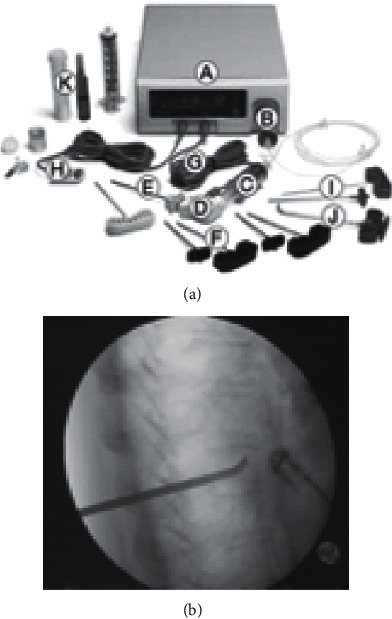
(a) Radiofrequency device and application system: (A) multiplex controller, (B) hydraulic assembly, (C) master syringe, (D) activation element, (E) locking delivery cannula, (F) StabiliT introducer-working cannula and stylet, (G) activation element cable, (H) hand switch cable, (I) straight line osteotome, (J) power curve navigating osteotome, and (K) StabiliT ER2 bone cement [52]. (b) Intraoperative X-ray of L1 vertebra (lateral view) using RFK [52].
